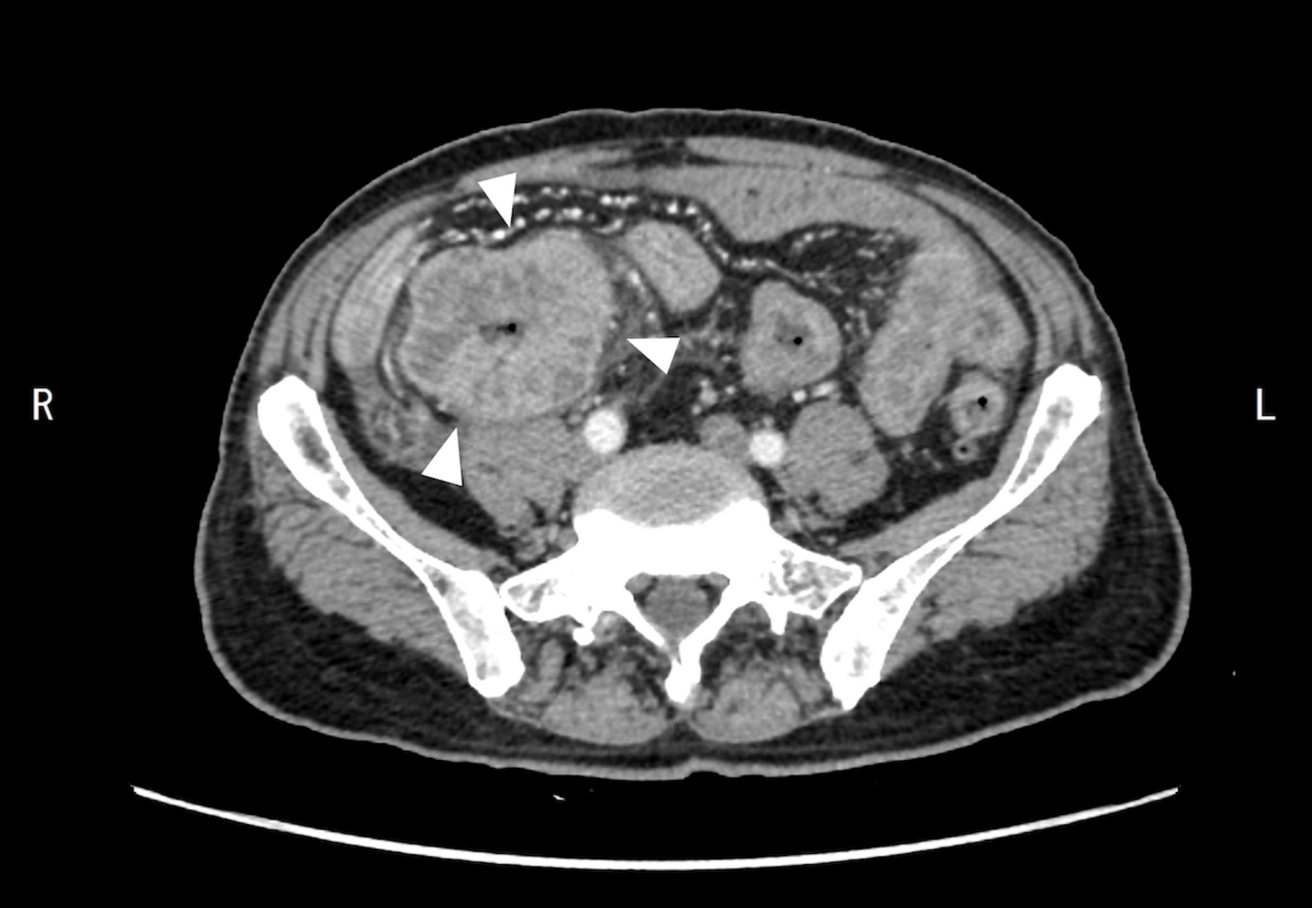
Abdominal computed tomography Abdominal computed tomography shows an ileocecal mass causing small bowel obstruction. The white triangles indicate the location of the tumor.
